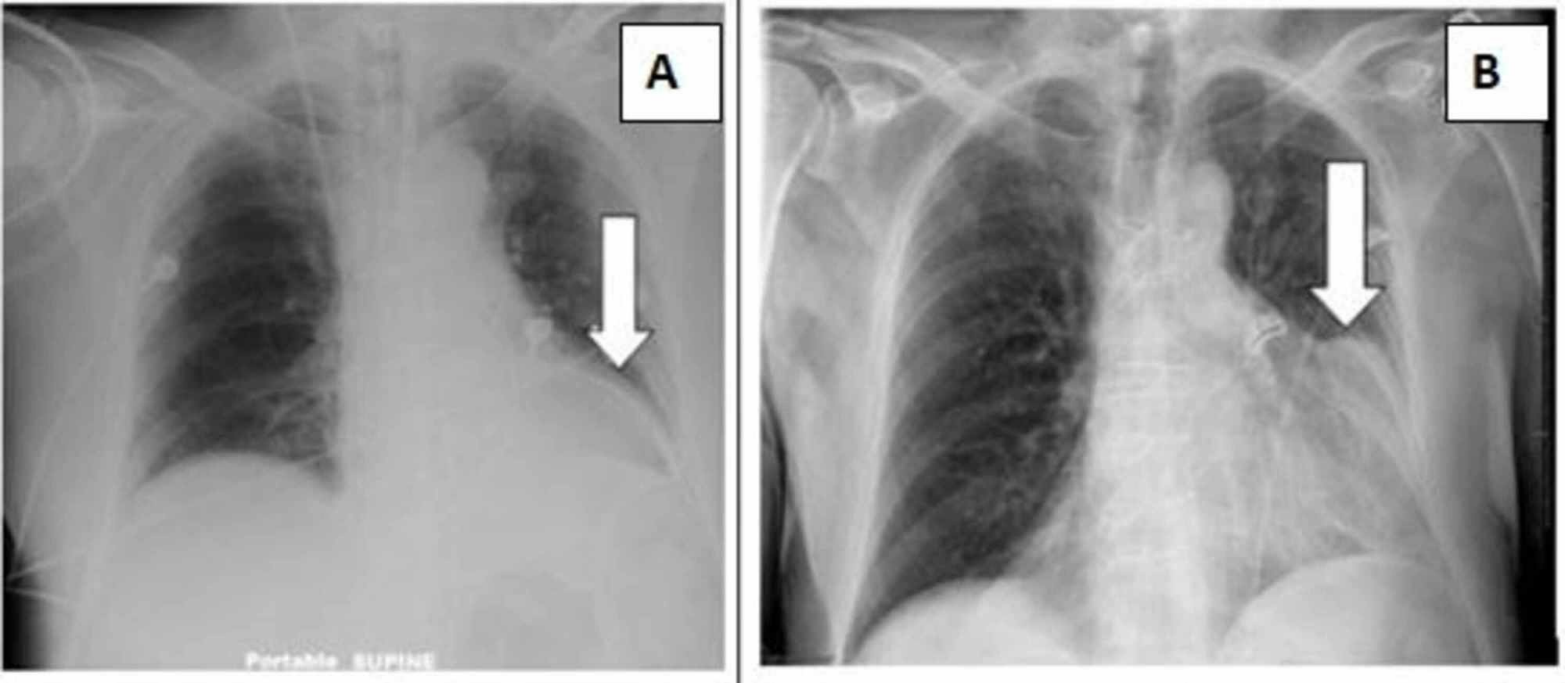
Chest X-ray during current hospitalization (B) compared with prior chest X-ray (A) Cardiomegaly is demonstrated by arrow in B
